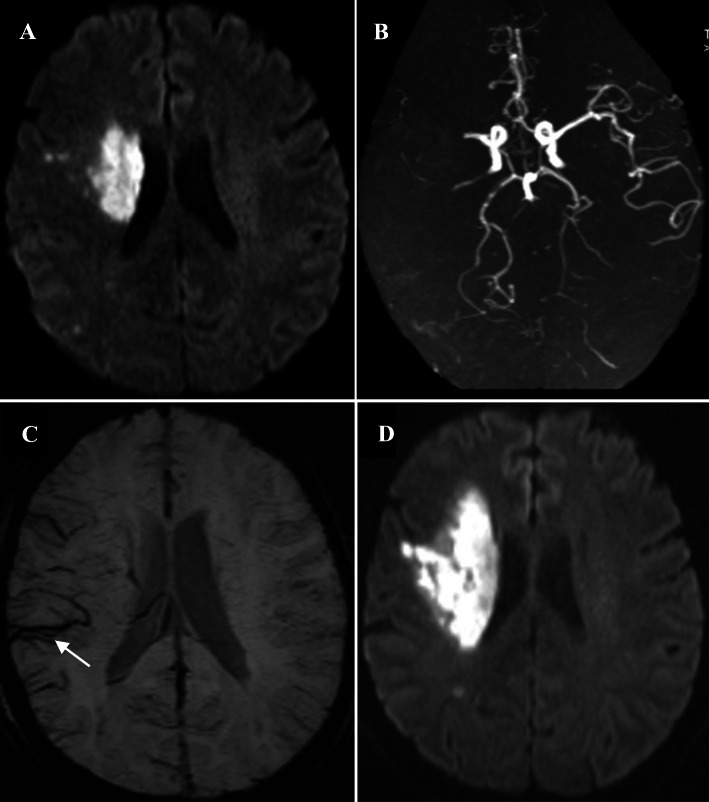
a Diffusion weighted imaging (DWI) in the first 24 h after admission: acute infarct in the right basal ganglia in one acute ischemic stroke patient with a National Institutes of Health Stroke Scale (NIHSS) score of 6 at admission. b Magnetic resonance angiography: occlusion in the right middle cerebral artery (MCA). c Susceptibility weighted imaging: asymmetrically prominent cortical vein sign in the right MCA territory (arrow). d Repeat DWI after early neurological deterioration (END), (Day 3 and the NIHSS score increased to 14): enlargement of the infarction
